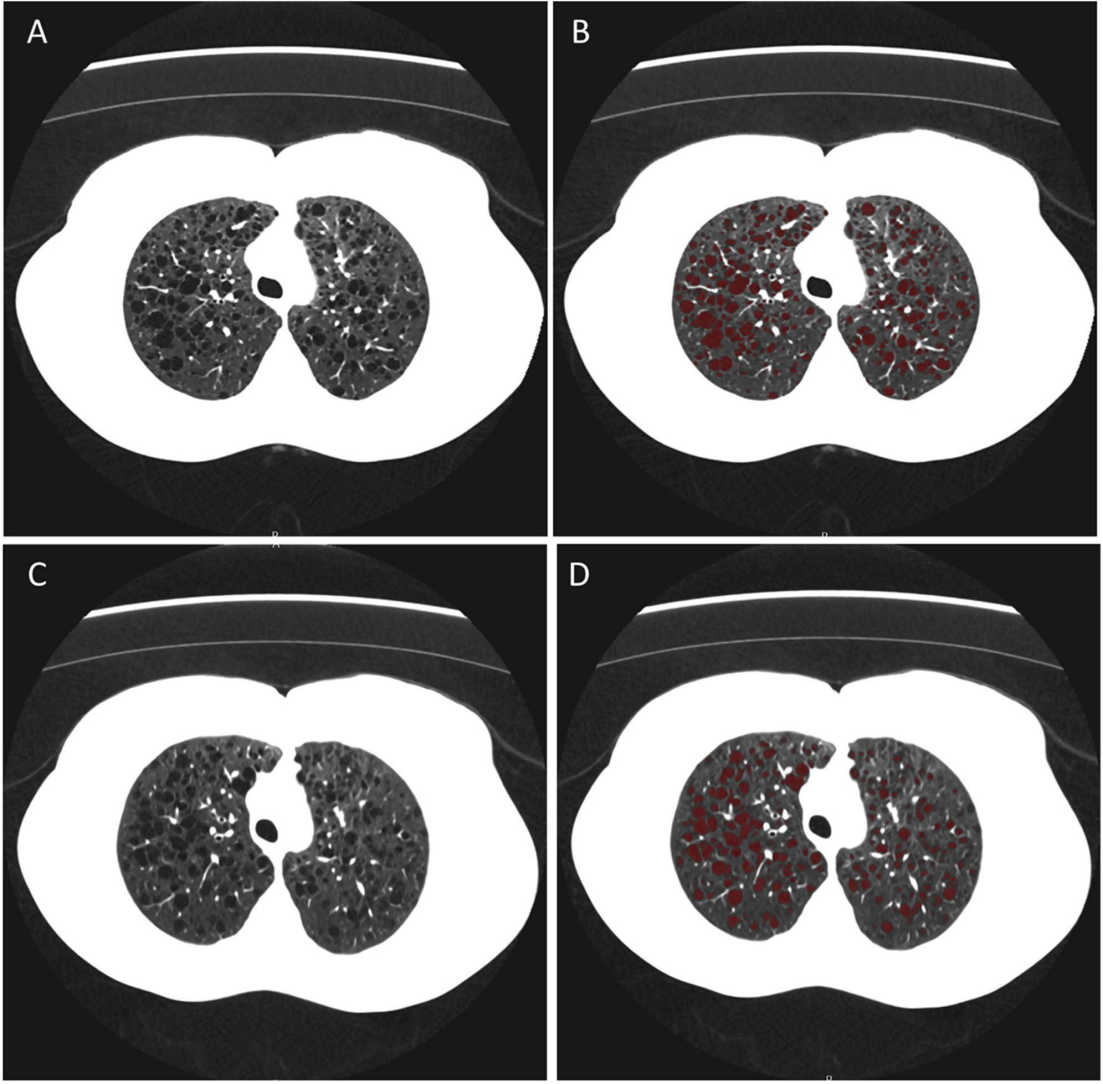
Chest CT of a 29-year-old female with LAM. A Standard scan with AIDR3D. B Standard scan with low attenuation areas highlighted in red. Radiation dose 1.90 mSv and cyst score 16.8%. C SilverBeam scan with AiCE. D SilverBeam scan with low attenuation areas highlighted in red. Radiation dose 0.33 mSv and cyst score 15.9%
